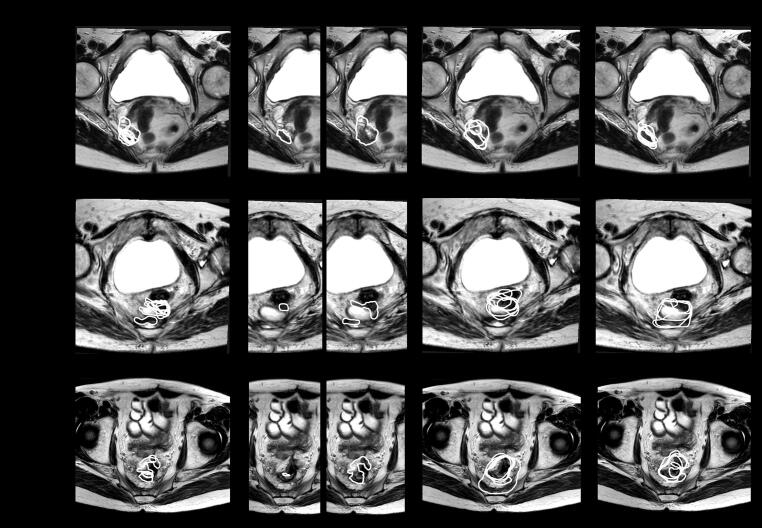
Three examples of recurrences with the constructed radiology contours and its effect on IOV. From left to right, MRI with contours by (a) radiologists, (b) median (left) and total (right) radiology contours, (c) GTV− contours and (d) GTV+ contours are shown for each case. From top to bottom, IOV decreased (case 1), remained the same (case 9) and IOV increased (case 14) from GTV− to GTV+.
